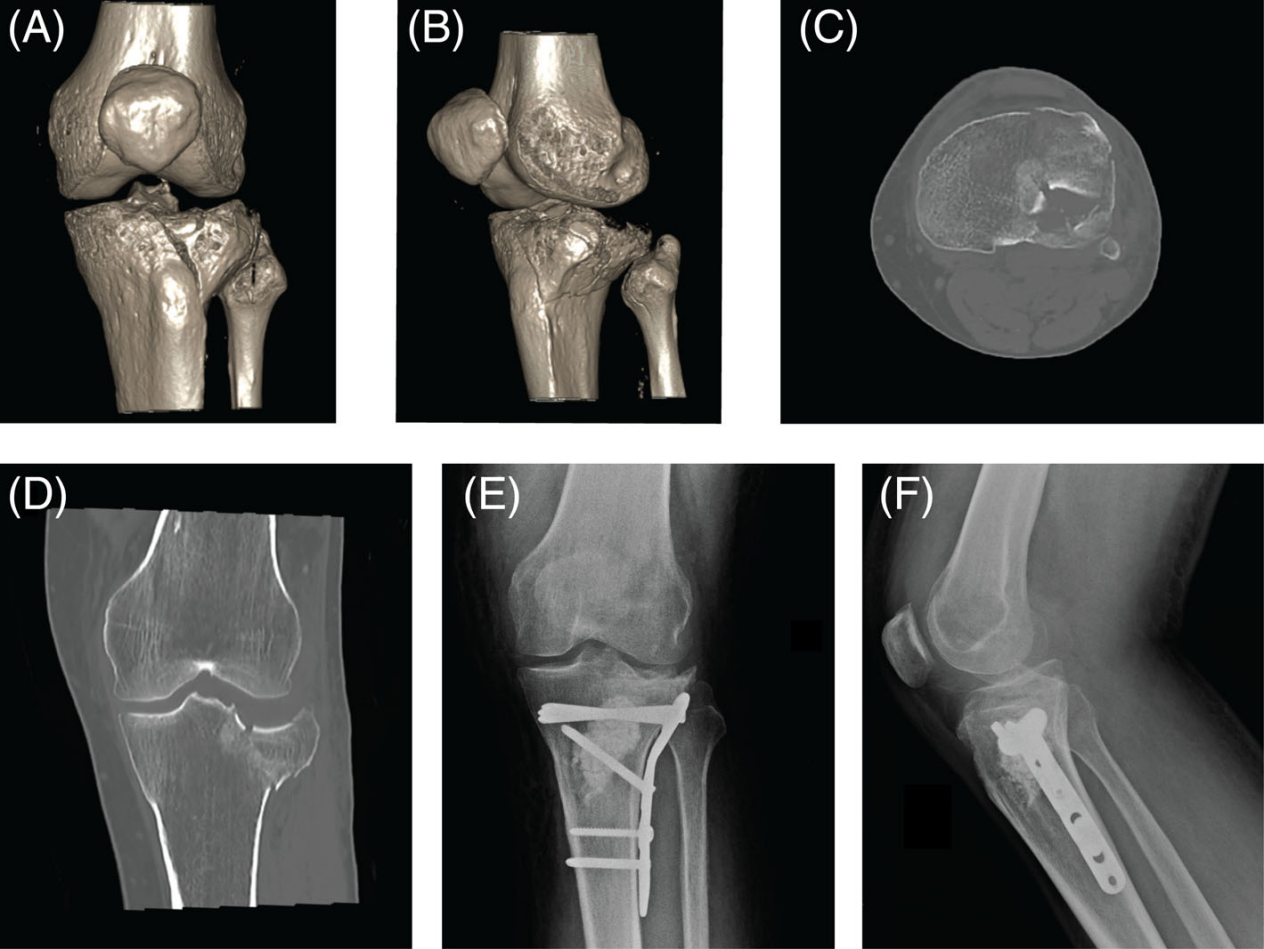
Case 3: A 46 year‐old‐male with an AO 41‐B3 fracture. (A, B) preoperative 3D‐CT scan reconstruction images; (C) CT axial image; (D) CT coronal view; (E, F) Anteroposterior and lateral radiographic images 6 months after surgery made with plate and screws and using artificial bone graft.
